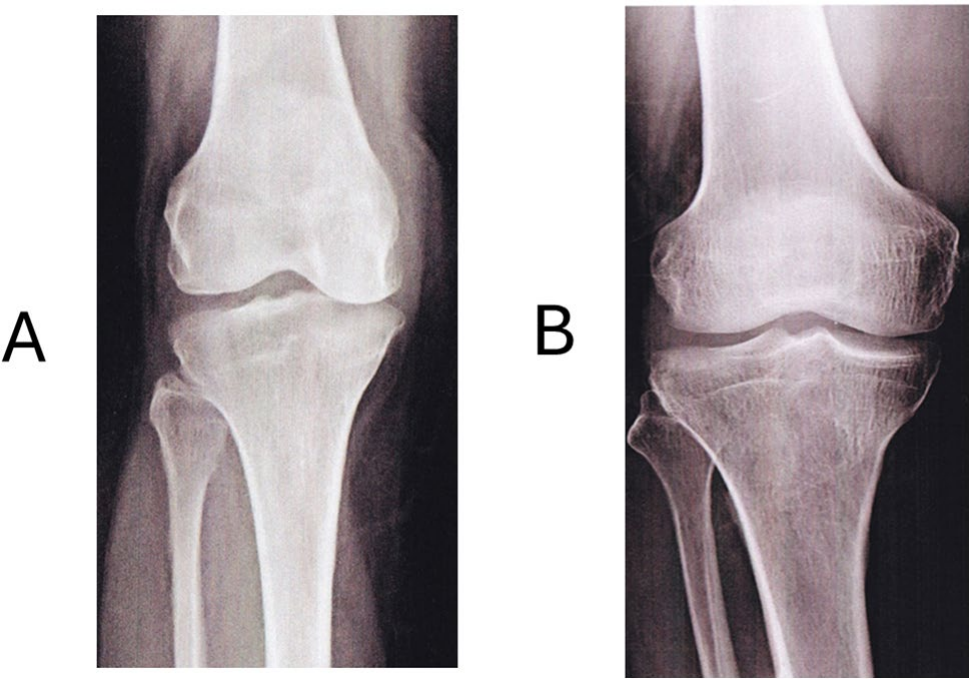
Representative radiological X-ray images of Participant #0104's Knee Before (A) and After (B) nutraceutical treatment.
